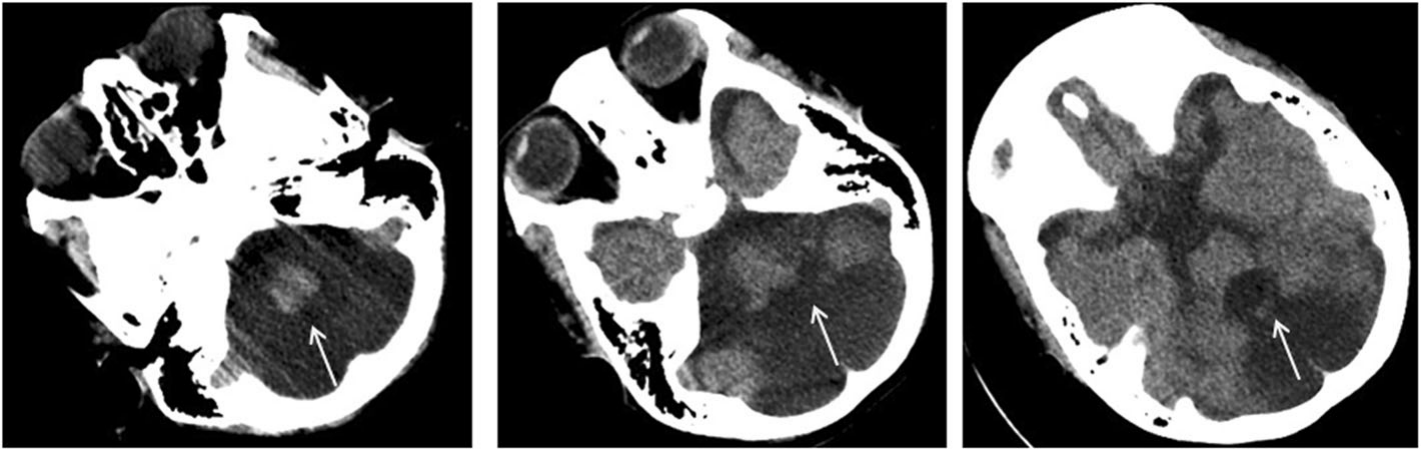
CT imaging showed the unclear of cerebellar vermis and enlarged fourth ventricle of the proband
